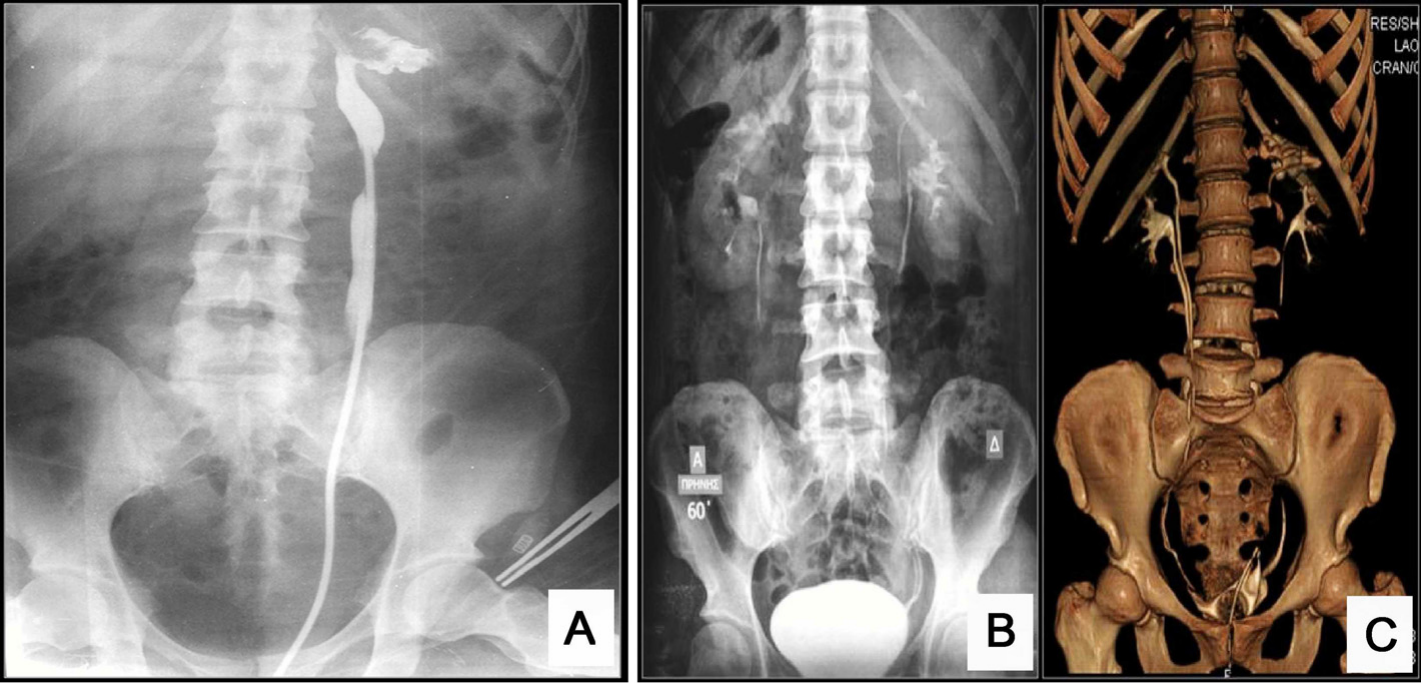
Intraoperative retrograde pyeloureterography via insertion of Nelaton catheter through the small opening of the intravaginal cystic ‘mass’. The upper half of a dilated ureter is opacified as well as a dilated pelvicalyceal system at the upper pole of the left kidney (A). Excretion urography at 60 minutes post-injection (B) and volume rendered computed tomography (CT) urography (C) bilateral duplication of pelvicalyceal systems and ureters. The upper pole of the left kidney drained by the ectopic ureter has a grossly dilated pelvicalyceal system (C). The ectopic left ureter is no longer dilated.
